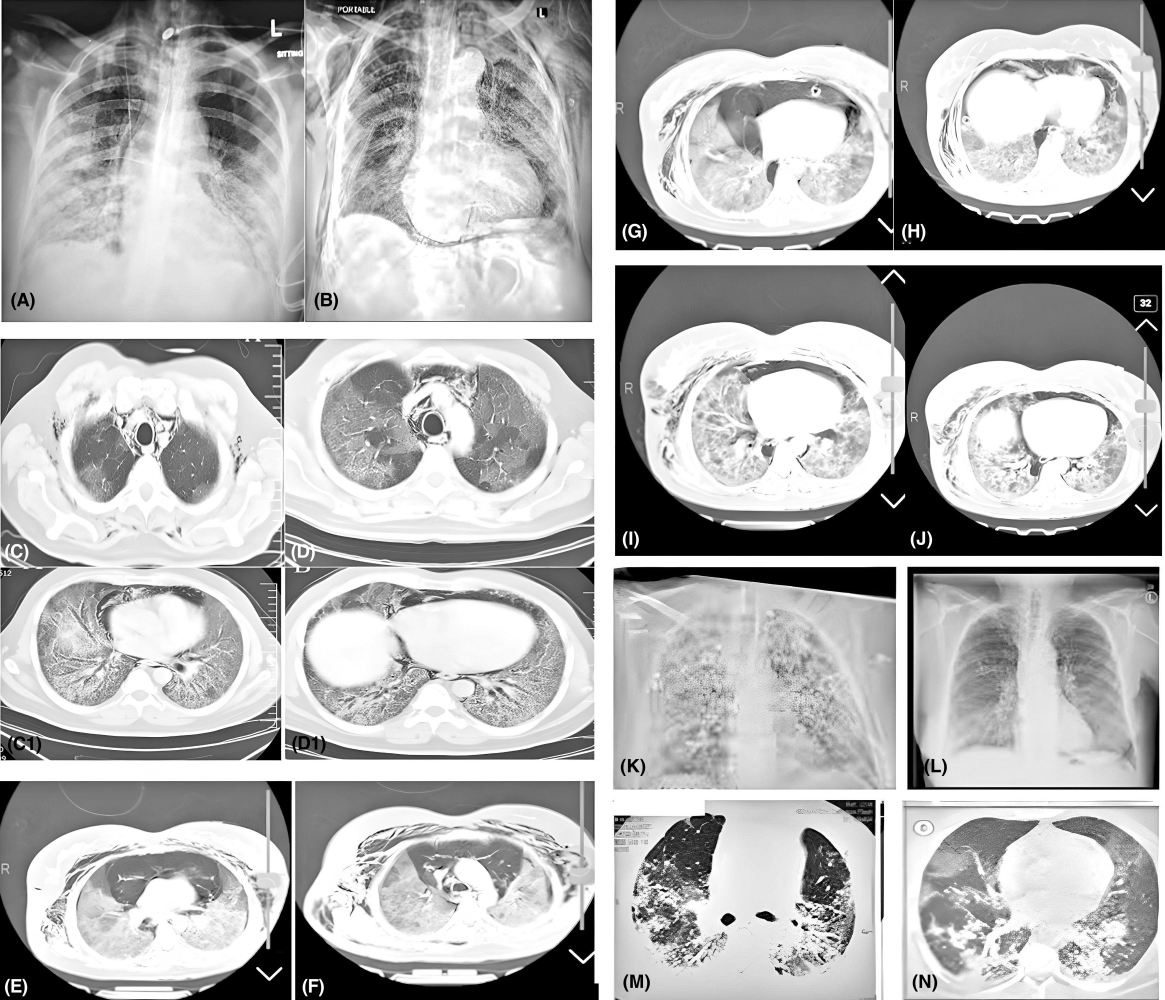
(A,B) Chest x‐ray on presentation with COVID‐19 pneumonia showing bilateral middle, upper, and lower zone infiltrates. (C,D and C1, D1) CT‐scan showing development of right and left side‐milled PT, PM. (E,F) CT‐scan of chest show tension pneumomediastinum, extensive SE and PT with compress of heart and mediastinum. (G,H) CT‐scan of chest show decreased the size of mediastinum and SE after chest tube and subclavicular incision. (I,J) CT‐scan of chest show decreased the size of mediastinum and SE after chest tube and subclavicular incision. (K,L) show CXR post 3 months after treatment. (M,N) CT‐scan of chest show post COVID 3 months after treatment.
